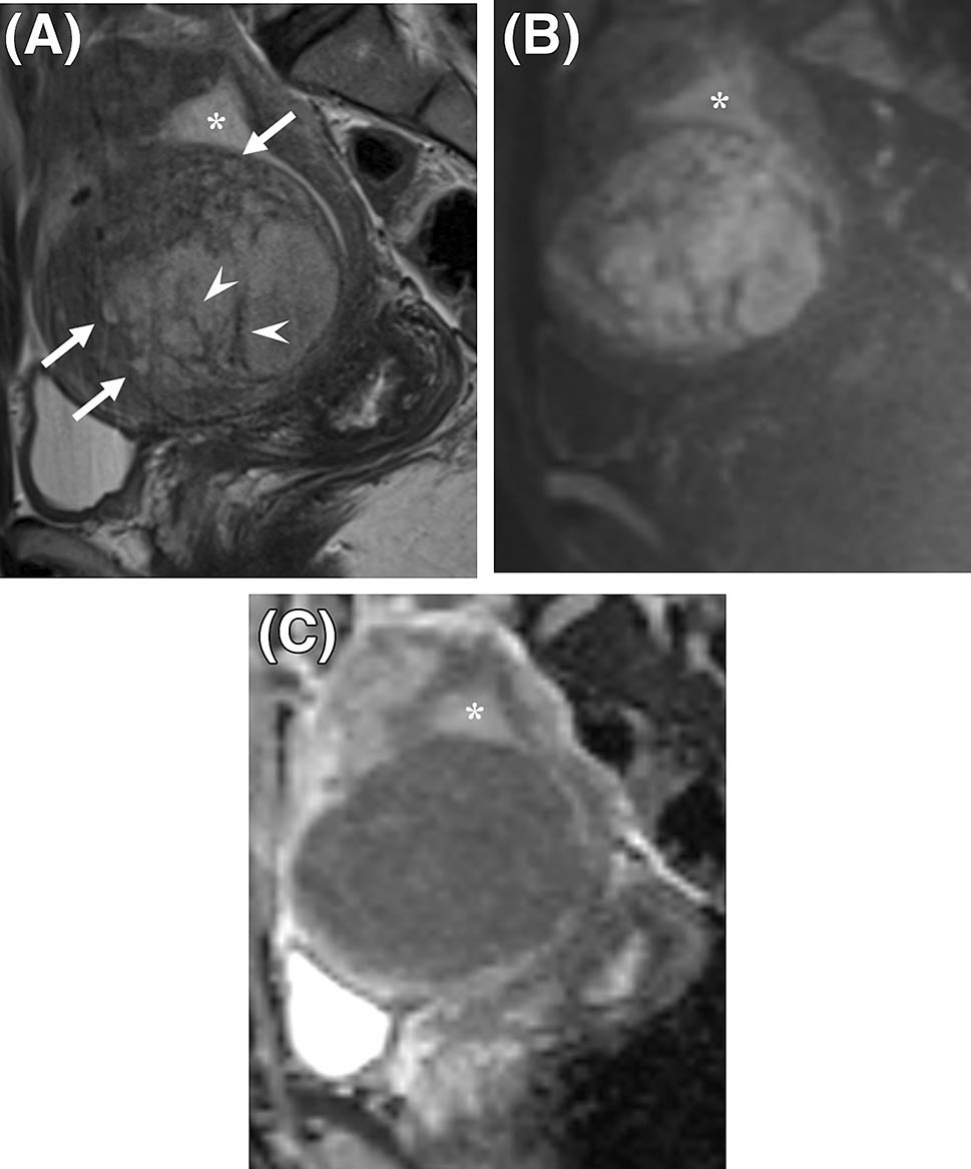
A representative case of low grade endometrial stromal sarcoma, showing worm-like nodular extension (A, arrows) and intra-tumoral low signal intensity (SI) bands (A, arrowheads) on T2-weighted images. The tumor shows high SI on diffusion-weighted images (B) with low apparent diffusion coefficient values (C). Asterisks were placed on endometrium (A–C).
